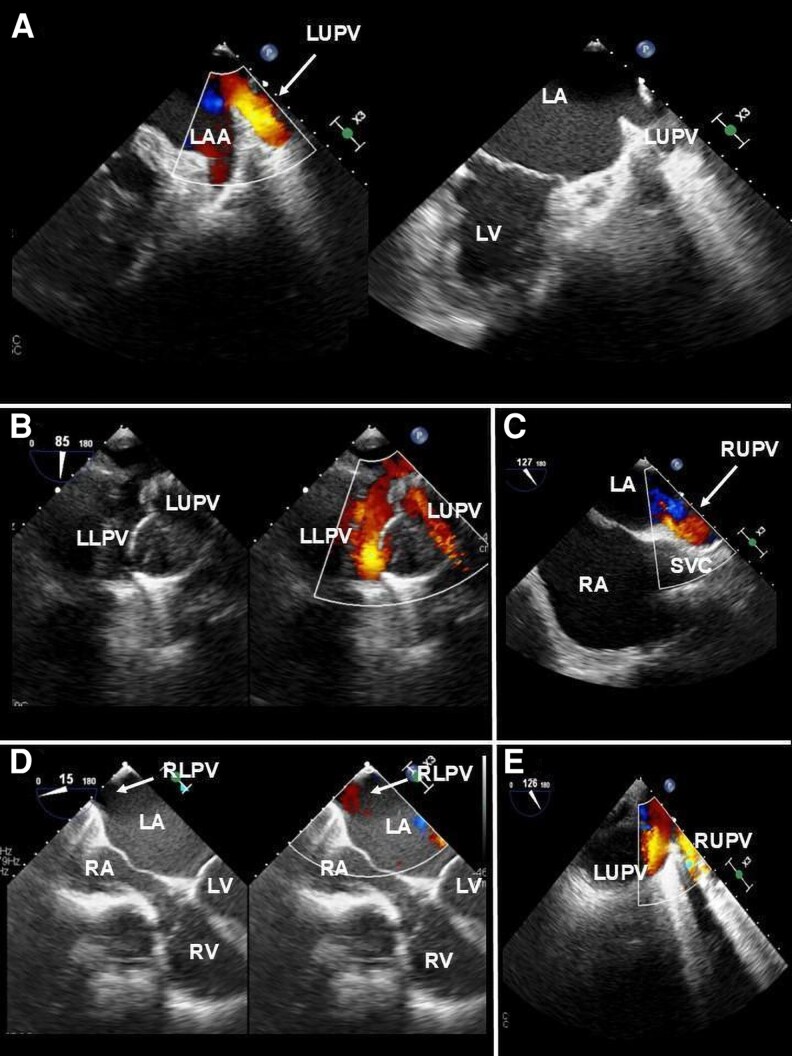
The TOE approach for visualization of PVs requires scanning from the ME level. (*A*): LUPV: from the ME 2-chamber view, the probe is rotated counterclockwise; slight withdrawal and sector rotation back toward ∼60° show the LUPV entering the LA supero-posterior to the LAA (‘LAA view’). (*B*): LLPV: advancing the probe from the LAA view, the LLPV becomes visible once the LAA disappears, with a change in Doppler flow direction. (*C*): RUPV: from the ME bicaval view, counterclockwise rotation of the probe reveals the RUPV entering the LA adjacent to the SVC (‘modified bicaval view’). (*D*): RLPV: from the ME 4-chamber view, clockwise rotation and probe advancement with sector rotation up to 30° demonstrate the RLPV entering the LA from an infero-posterior position. (*E*): RUPV and LUPV: visualized together in a ME long-axis view (∼120°) with extreme counterclockwise rotation, slight probe withdrawal, and probe tilting. LA: Left atrium; LAA: Left atrial appendage; LLPV: Left lower pulmonary vein; LUPV: Left upper pulmonary vein; LV: Left ventricle; ME: Mid-oesophageal; PV: Pulmonary vein; RA: Right atrium; RLPV: Right lower pulmonary vein; RUPV: Right upper pulmonary vein; SVC: Superior vena cava; TOE: Transoesophageal echocardiography.
